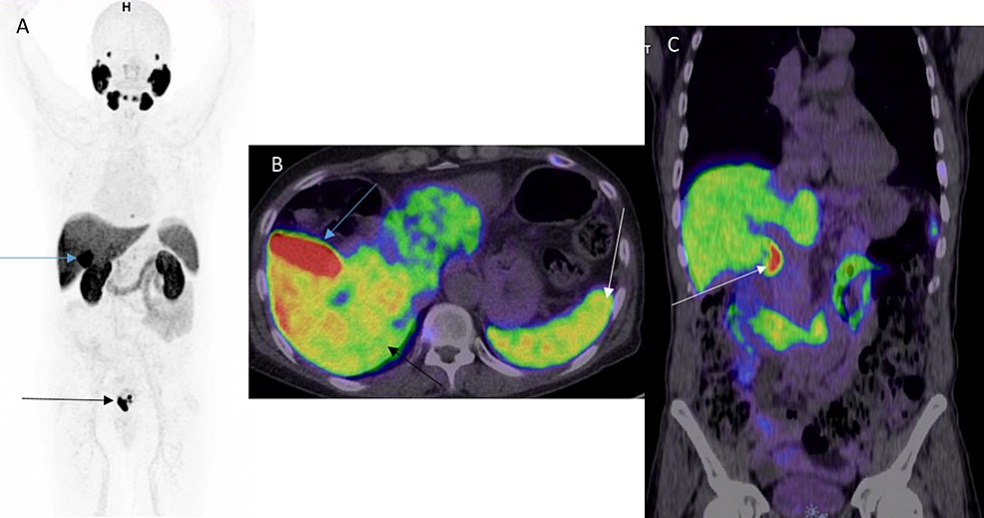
Normal distribution in the gallbladder and bile duct A: 68Ga PSMA MIP image shows physiological activity in the gallbladder (blue arrow). Note the cancer recurrence in the prostatic bed (black arrow). B: Axial image of the upper abdomen shows intense physiological activity in the gallbladder (blue arrow). Moderate uptakes are seen in the liver (black arrow) and spleen (white arrow), which are physiological. C: Coronal image of the chest and abdomen shows intense physiological activity in the common bile duct (white arrow). The uptake correlates to the bile duct on CT (not shown).
